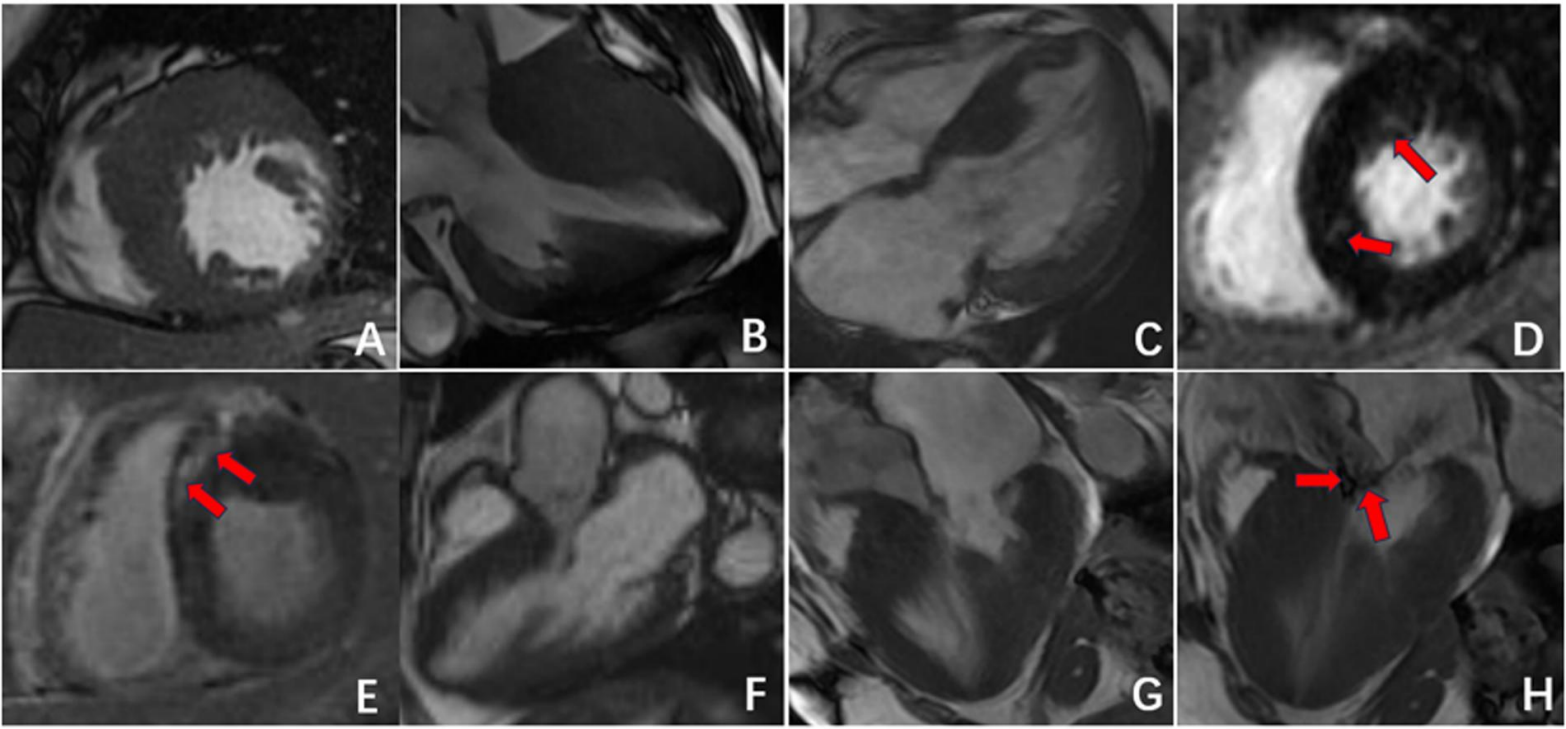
Radiographic findings of HCM. (A–C) Asymmetric myocardial hypertrophy; (D,E) mid-myocardial enhancement (red arrow); (F–H) HCM resulting in signs of an outflow tract with a systolic high-velocity blood flow signal (red arrow).
